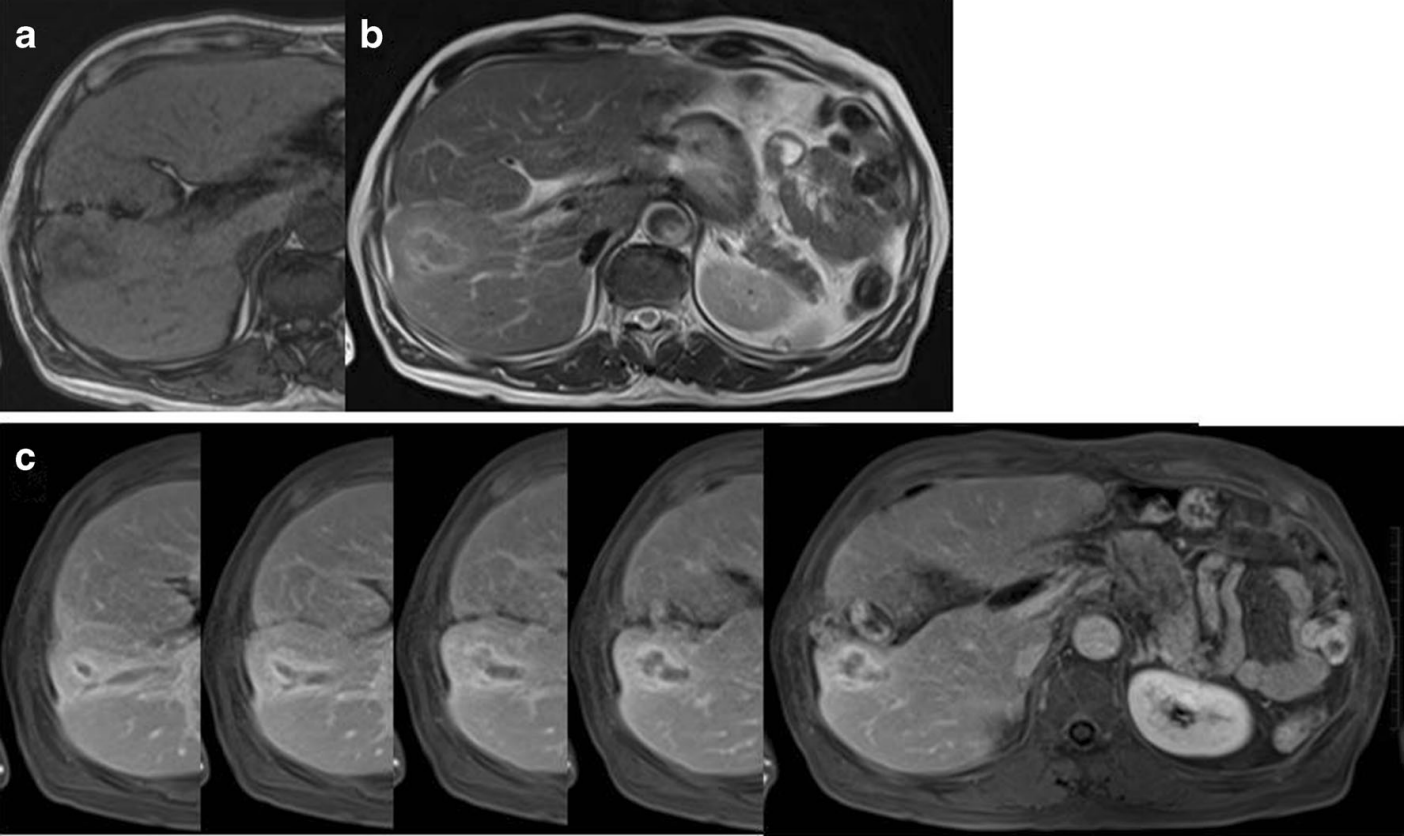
Abdominal contrast MRI images of the liver tumor at the initial visit to our hospital in August 2009. a T1W1. b T2W2. c Dynamic study
